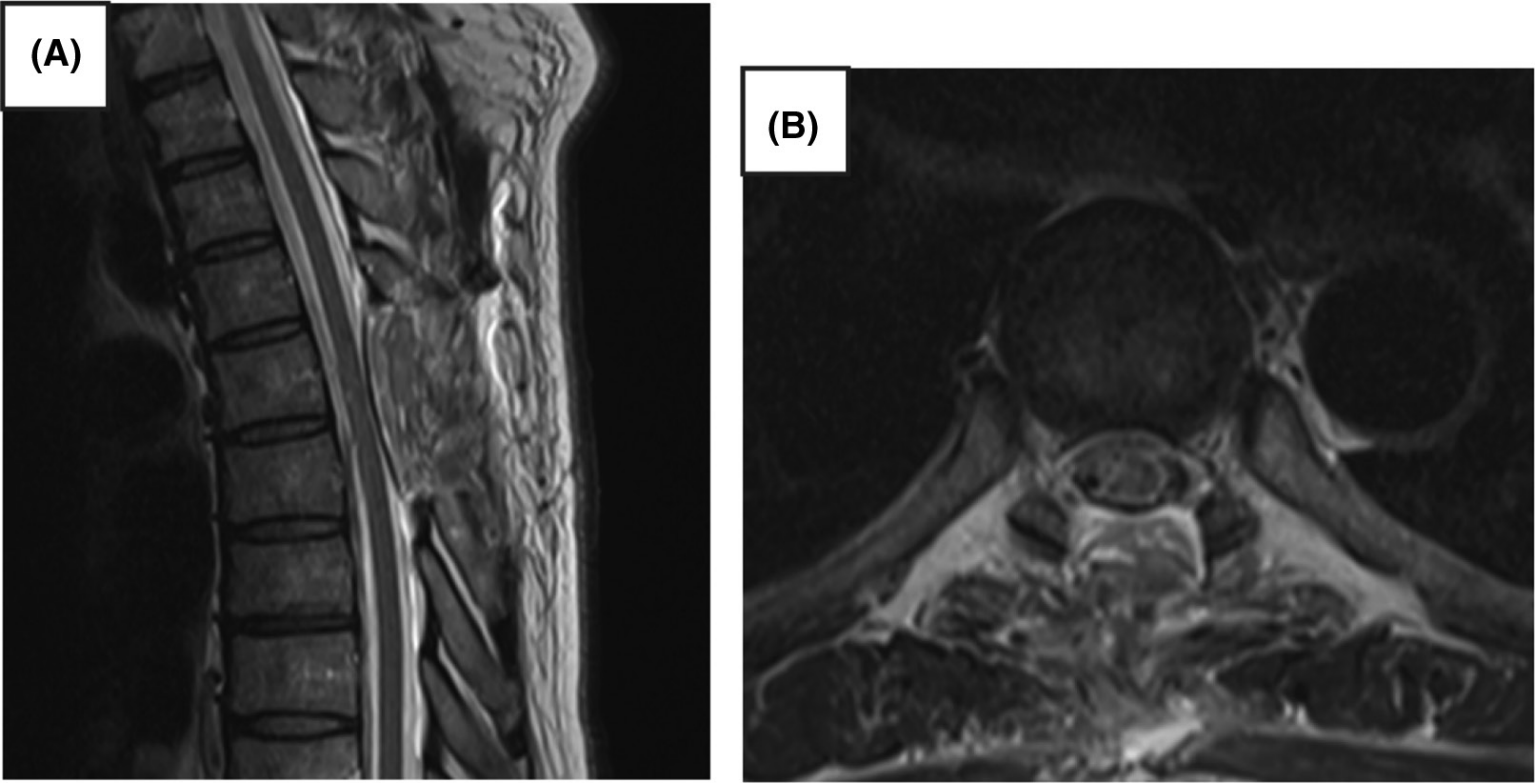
T2‐weighted magnetic resonance image obtained at 1‐week postoperatively, showing the normal position of the spinal cord: (A) Sagittal, (B) Axial.
